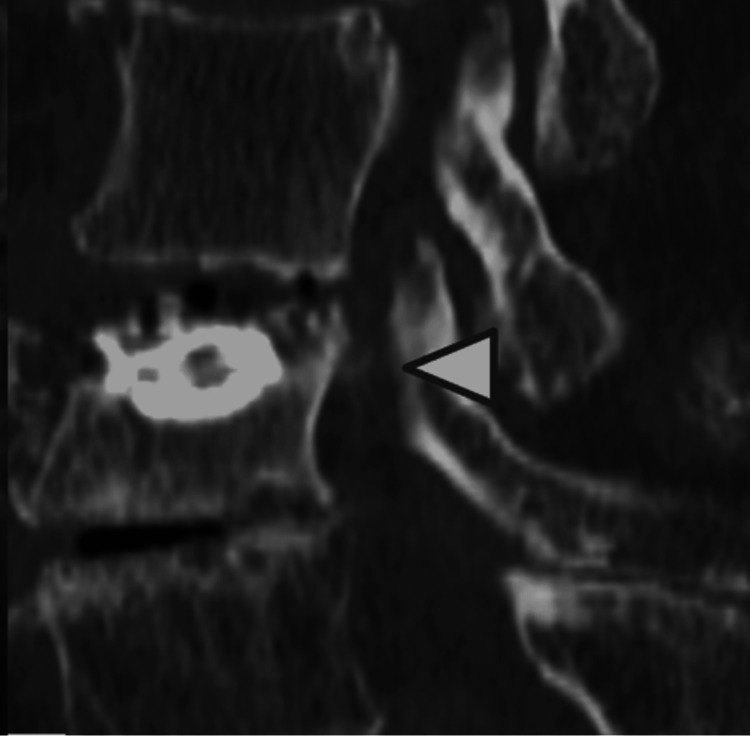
Sagittal view of postoperative 3D-CT Postoperative 3D-CT revealed a reduction of the bone fragment (white arrowhead).
